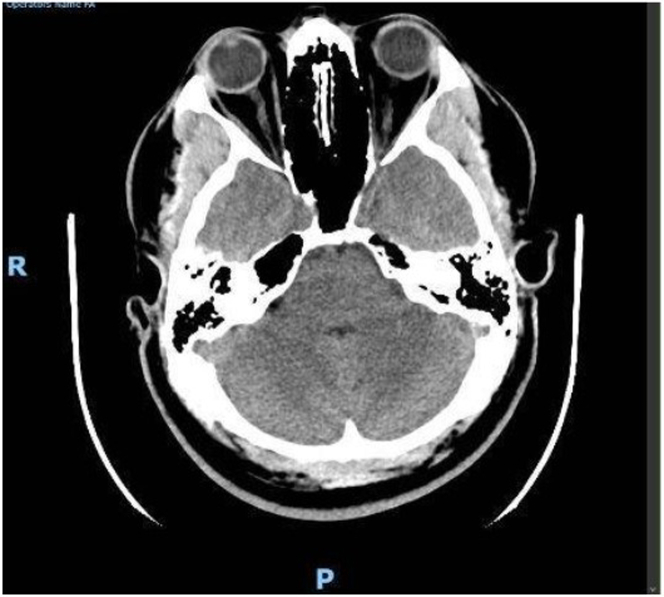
Head Computed Tomography (Noncontrast) Axial noncontrast computed tomography scan of the brain showing no acute intracranial hemorrhage, infarction, or mass effect.
